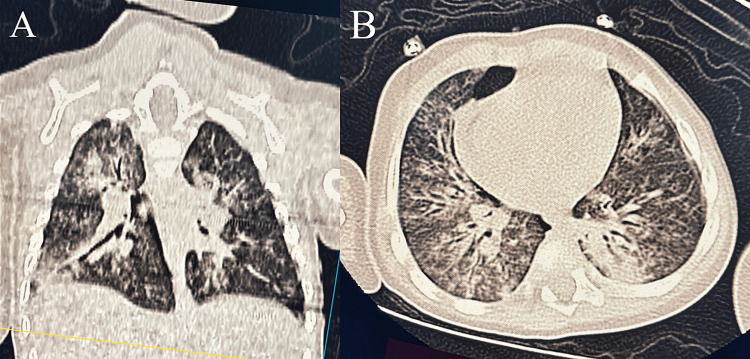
Coronal and axial views of the C+ chest CT. (A) Coronal CT image showing bilateral diffuse pulmonary infiltrates, consolidation, and peribronchovascular thickening. (B) Axial CT image showing bilateral diffuse confluent ground-glass nodules.
